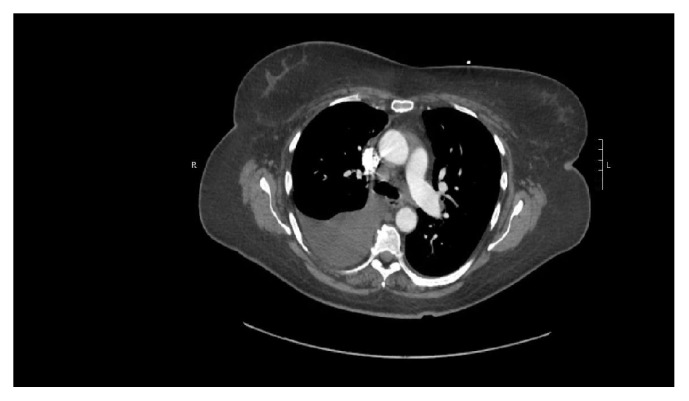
CT of chest showing mediastinal lymphadenopathy with presence of pleural effusion.
